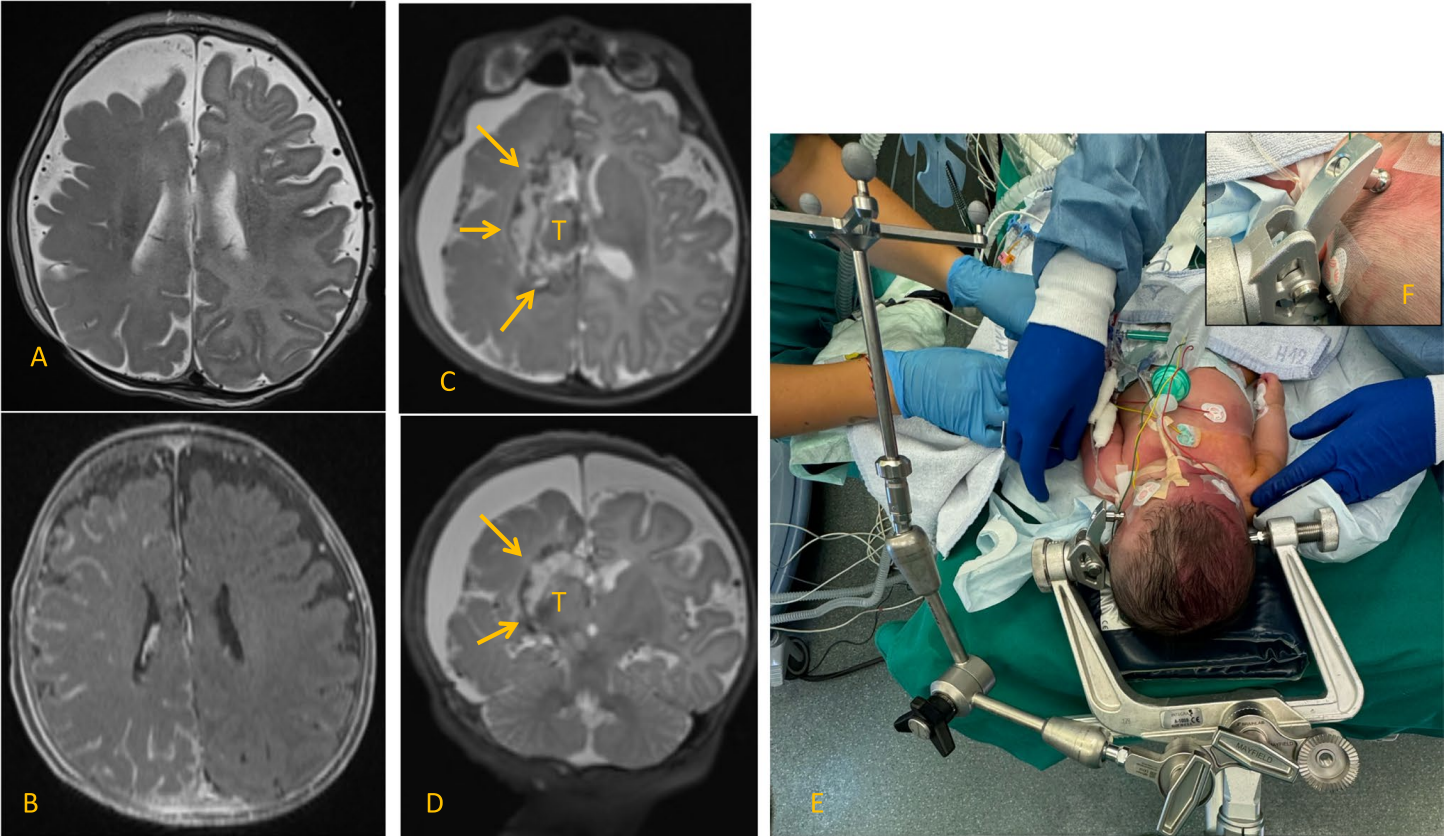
A T2 MRI scan showing the developmental delay of the right hemisphere with no gray white differentiation. B T1 gadolineum scan with diffuse meningeal enhancement of the complete right hemisphere as the typical appearance of meningeal angiomatosis Sturge-Weber. C Postoperative axial T2 MRI scans demonstrating the isolated thalamus on the right side as a result of the minimal invasive functional hemispheric disconnection procedure, arrows demonstrating the disconnection line, T: thalamus. D Postoperative coronar T2 MRI scan showing the complete disconnection of the right hemisphere, arrows demonstrating the disconnection line, T: thalamus. E Positioning of the infant on a gel pad on the OR table. F Head arrested with kids pins on the skull base to overcome the instability of the skull with still open fontanels. Navigation mounted at the skull clamp (Brainlab, Munich, Germany)
